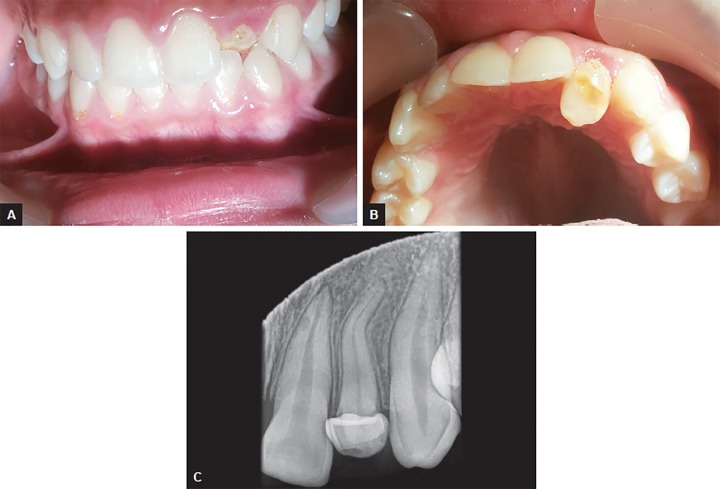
(A) Anterior crossbite in relation to #22, (B) crown dilaceration of #22, (C) periapical radiograph showing crown-root dilaceration of #22
